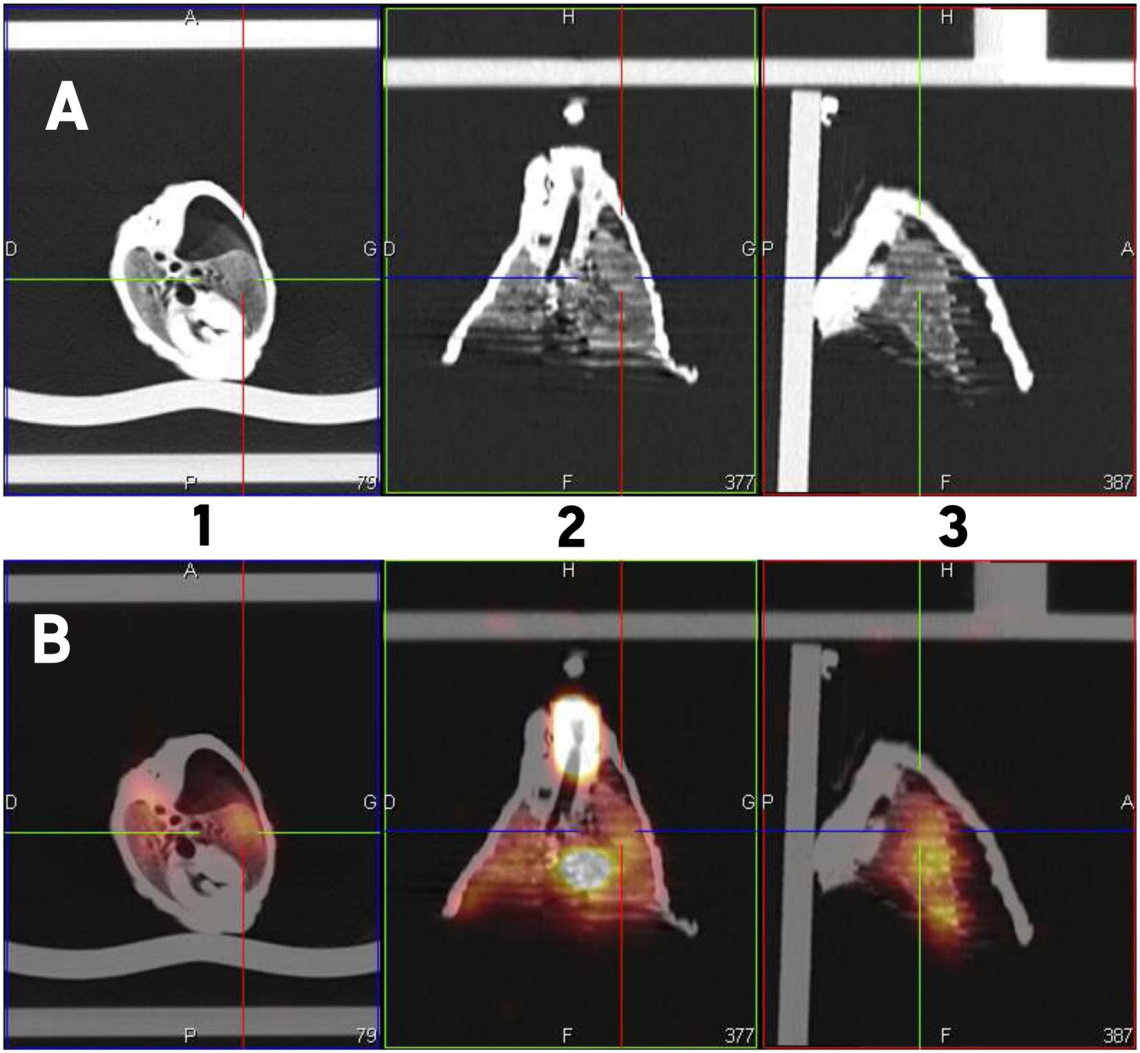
Diethylene triamine pentaacetic acid (^99m^Tc-DTPA) images after three dimension reconstruction of the *ex vivo* model developed in this study. (A) tomography images. (B) fusion of tomography and scintigraphic images. 1: transversal view. 2: coronal view. 3: sagittal view.
